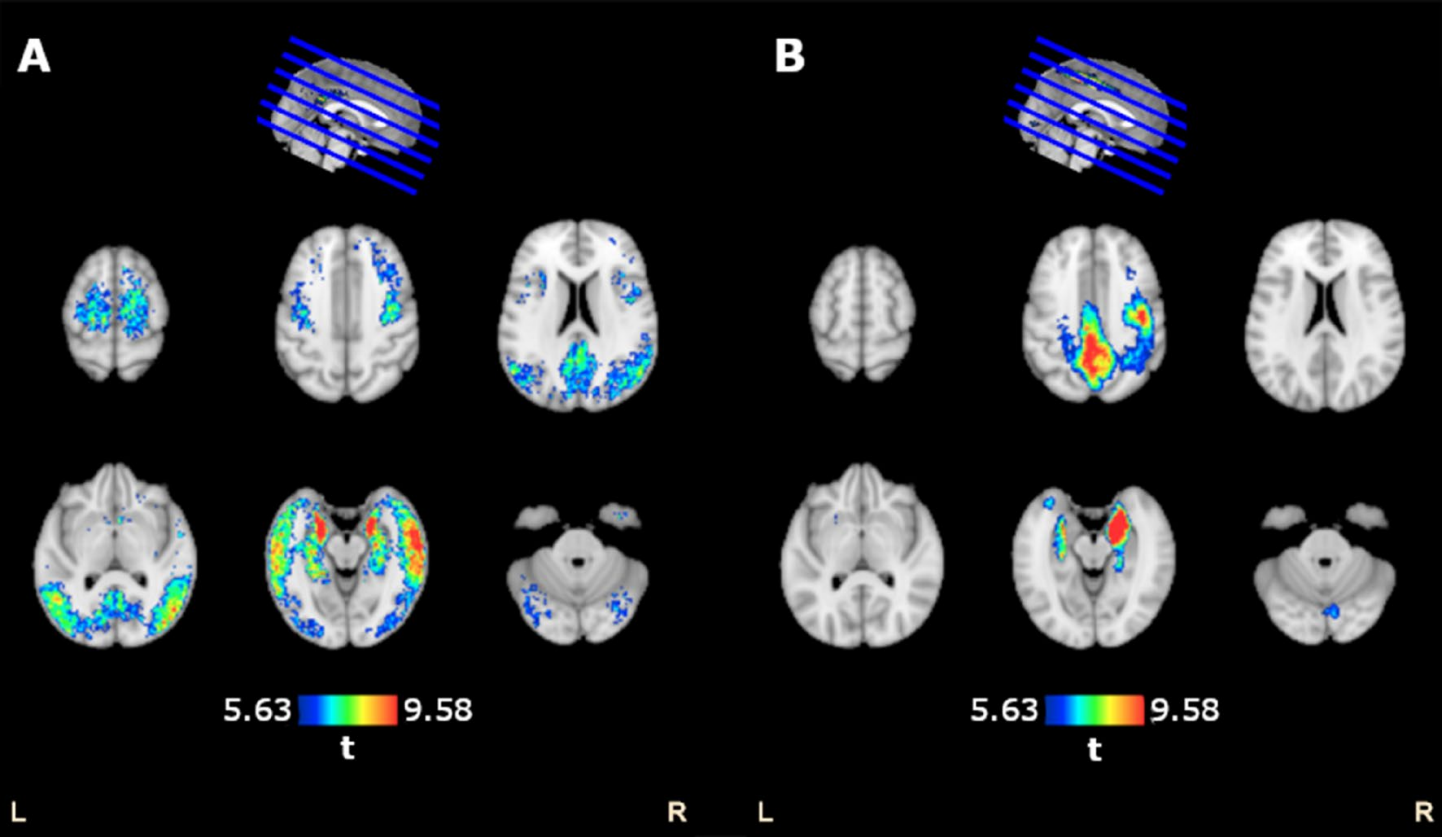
Comparison of SUVR-based and CNN-based GLM analyses. (A) Tau-PET SUVR-Aβ CL GLM significant clusters (FWE *p* < 0.05; cluster extent of 200; covaried for age and sex) and (B) CNN-based saliency-Aβ CL significant clusters (FWE *p* < 0.05; cluster extent of 200; covaried for age and sex).
